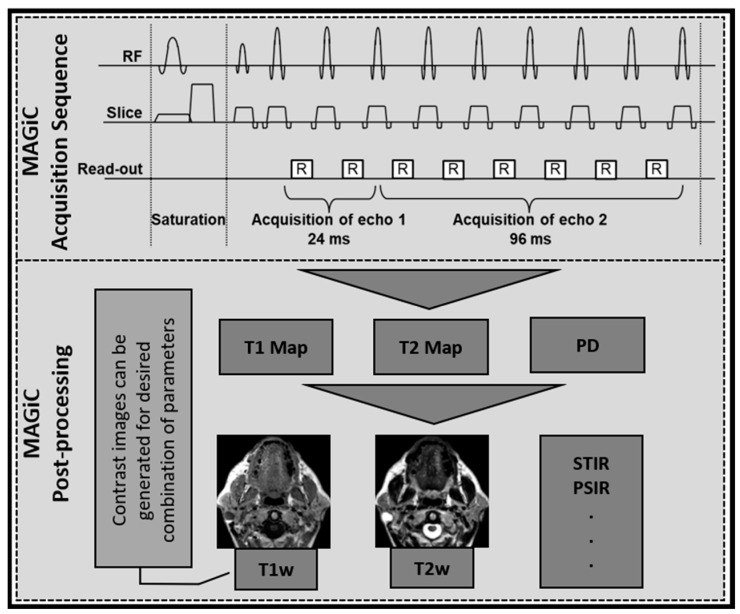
Synthetic MRI acquisition and post-processing: one representative block of MAGiC sequence, and MAGiC post-processing consist of generating quantitative and qualitative images. Qualitative images are generated using the relaxometry and proton density (PD) maps for the desired combination of parameters. Representative T1 and T2 images are shown, and additional contrast images can also be generated.
